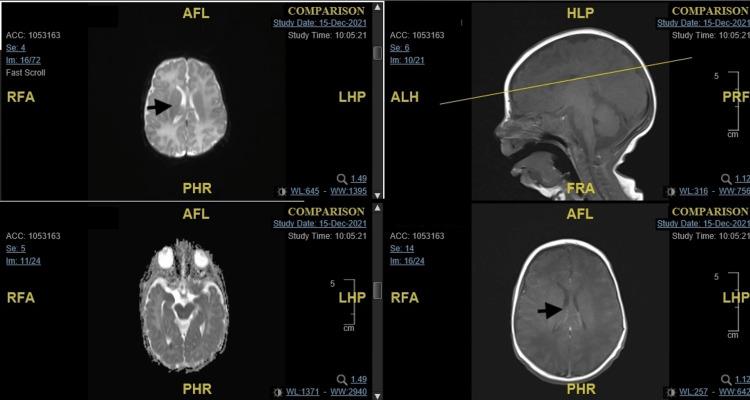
Magnetic resonance imaging showing ventricular enlargement with no signs of meningitis.
